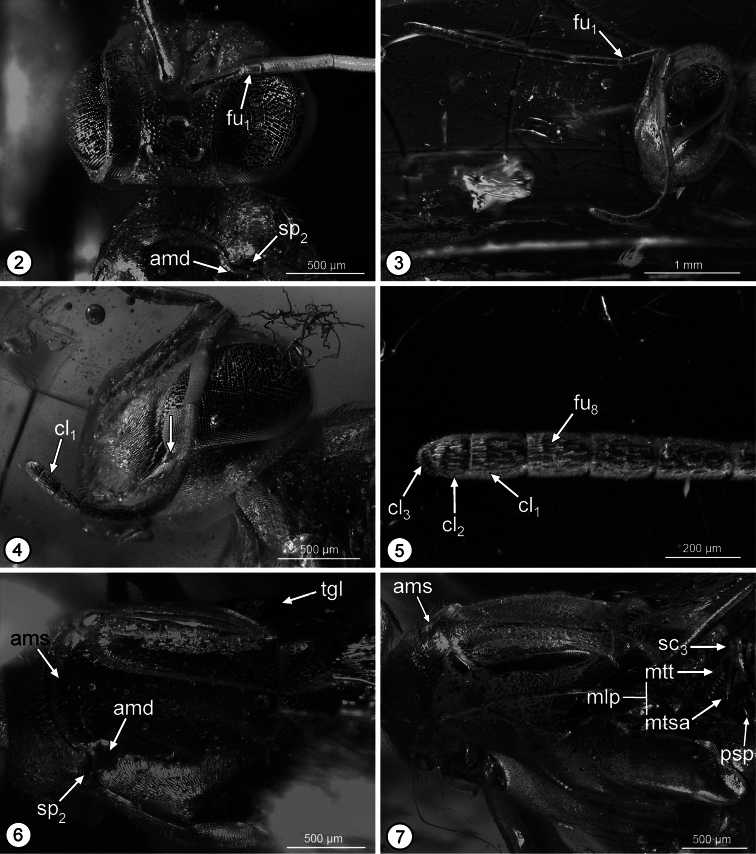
Propelma rhodendorfi*: 2 head, frontodorsal view 3 head and antennae, lateral view 4 head and right antenna, lateral view 5 apical three funiculars and clava 6 mesoscutum, dorsal view 7 mesosoma, dorsolateral view. See Methods for abbreviations for structural features (arrow on Fig. 4 points to triangular region differentiated by putatively bifurcate malar sulcus).
